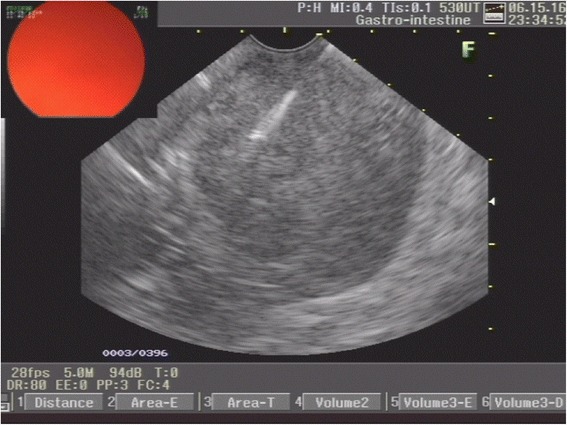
Endoscopic ultrasound-guided fine needle aspiration of the subepithelial lesion originating at the fourth layer (muscularis propria)
